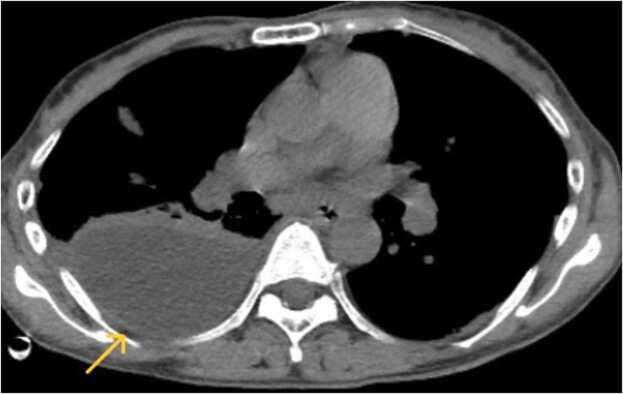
Follow-up CT on day six after hospitalization. That revealed residual pleural effusion in the right posterior space (yellow arrow), which had increased.
